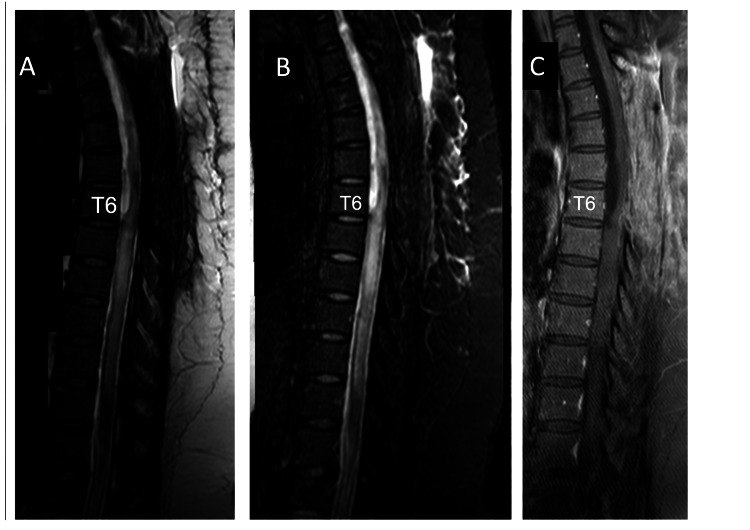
Sagittal T2-weighted (A), T2-STIR (B), and T1-weighted (C) MRI, taken one month after the first decompressive laminectomy from T3 to T6 levels, show a reduction of cystic compression and the presence of spinal cord edema from T6 to T11 levels.
